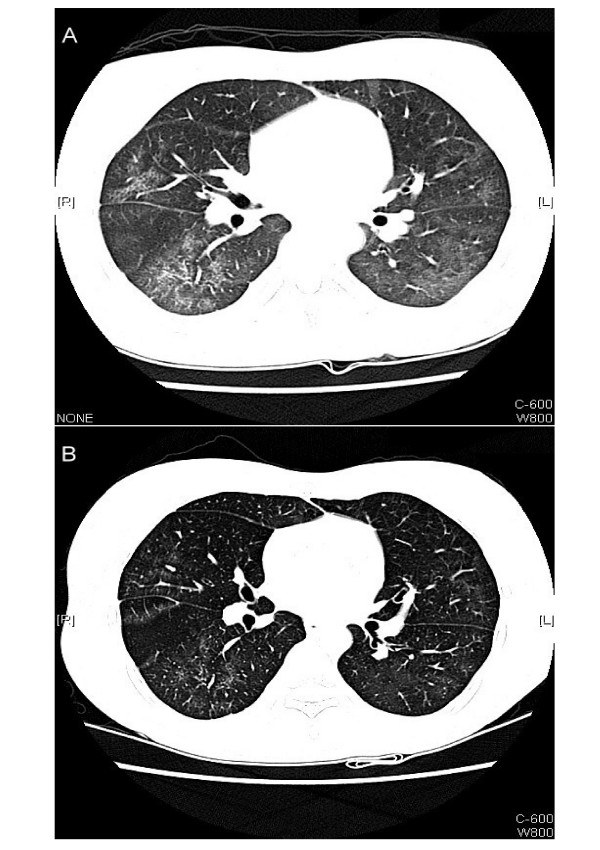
Residual abnormality on HRCT of a SARS patient with high HRCT score at 60 days (A). HRCT became almost normal at 90 days (B).
